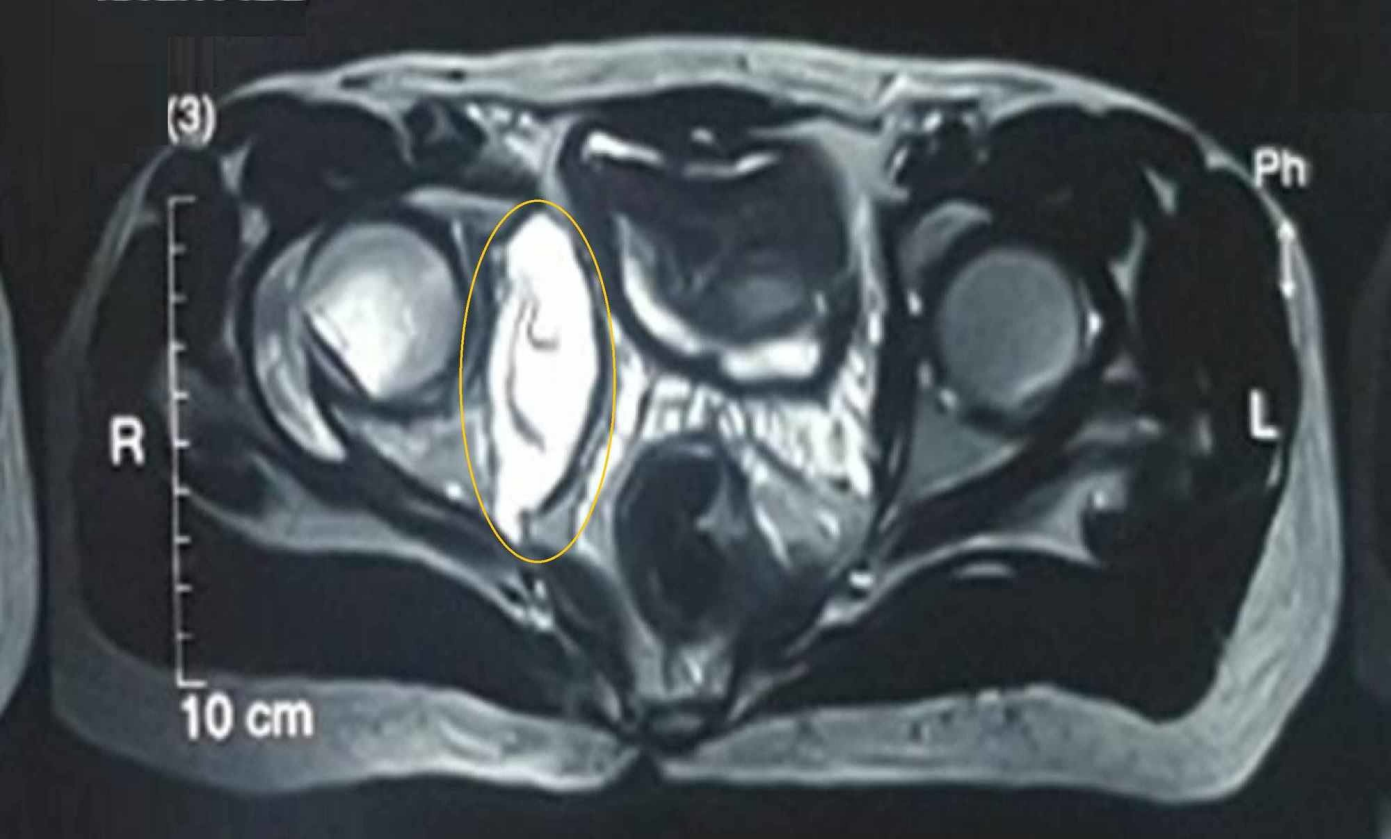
Magnetic resonance imaging of pelvis showing a homogeneous hyperintense lesion on T2-weighted image in the right iliac blade, right sacroiliac, and hip joints A hypointense rim at the periphery of the cystic lesion with the “water-lily sign” was noted (circle).
